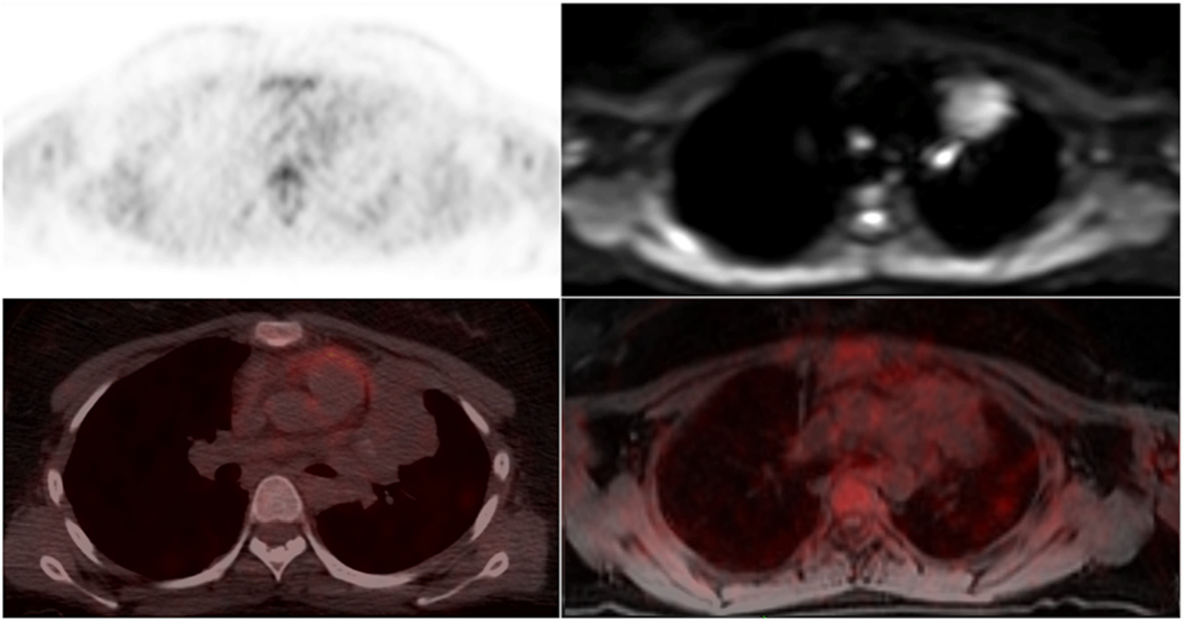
A male patient with Hodgkin’s disease stage IIIE. PET/CT/MRI after two cycles of chemotherapy. Top: Axial PET shows very faint uptake in the anterior mediastinal lesion; axial WB-DW-MRI (b value = 800) shows restricted diffusion (calculated ADC_mean_ = 0.96 × 10^−3^ mm^2^/s). Bottom: FDG-PET/CT and FDG-PET/MRI show a residual mediastinal mass without significant FDG activity. FDG-PET/CT and FDG-PET/MRI after the end of treatment confirmed complete response
